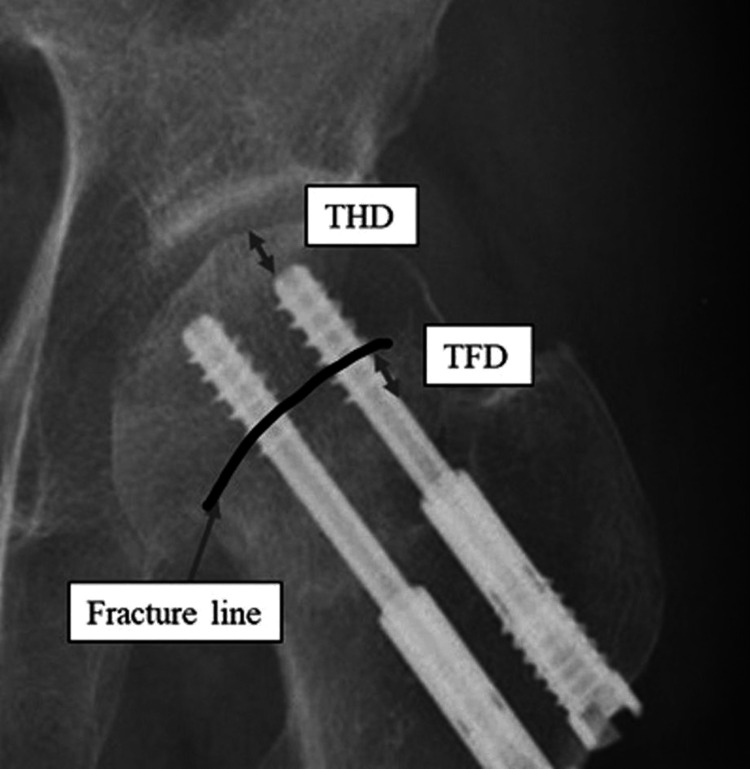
Postoperative X-ray: the TFD and THD were measured. Arrow shows the fracture line TFD: thread-fracture line distance, THD: thread-head cortex distance
